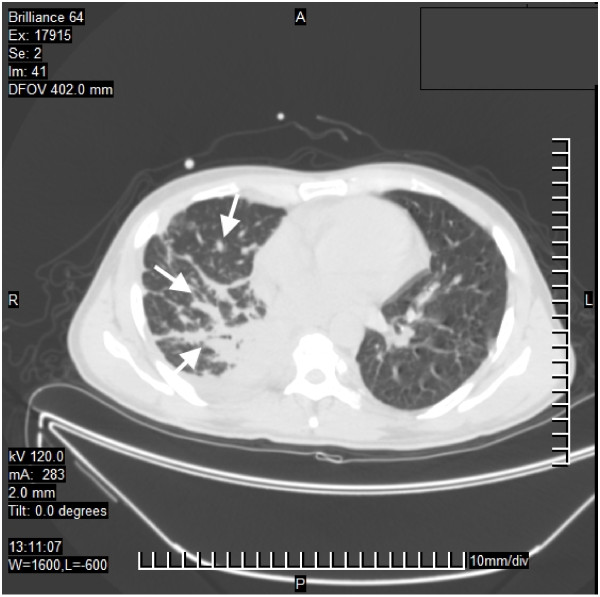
Computed tomography image of lung infection by *Mycobacterium abscessus *subsp. *bolletii*. The white arrows show parenchymal consolidation in both lungs, predominantly in the right side, and patchy ground-glass appearance with nodules.
